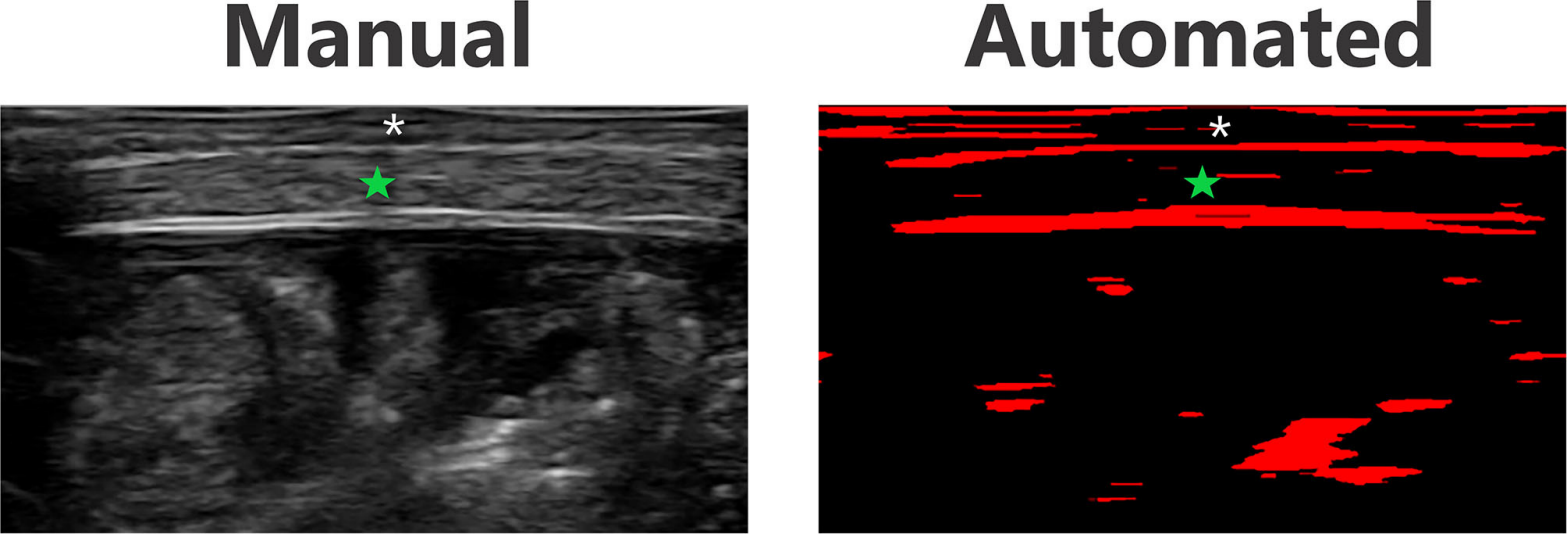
Ultrasound and automated MuscleSound, Inc. software image example of the lateral abdomen in the same subject. Green star—muscle layer. Asterisk—subcutaneous tissue layer.
